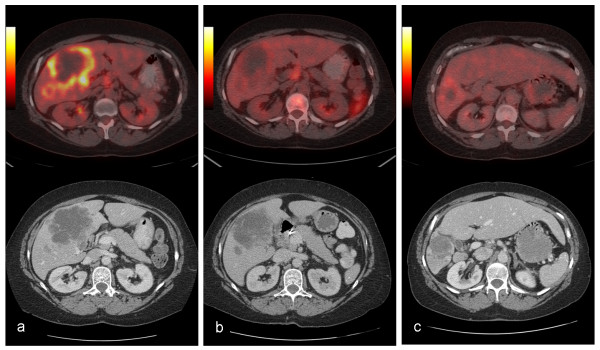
FDG-PET/CT image sets demonstrating progressive decrease in the functional and anatomic volume of the tumor with concurrent left lobe hypertrophy. a: Pre-treatment, b: 4-weeks after first SIRT treatment, c: At the completion of the full course of the treatment.
